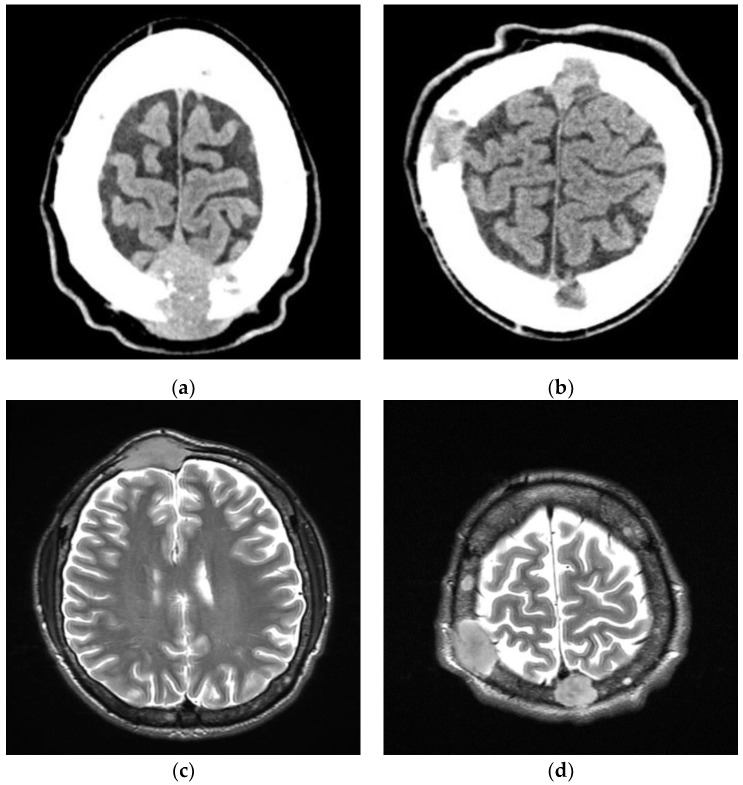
CT (a,b) and MRI (c,d) scans of plasma cell tumor infiltrating central nervous system (CNS).
